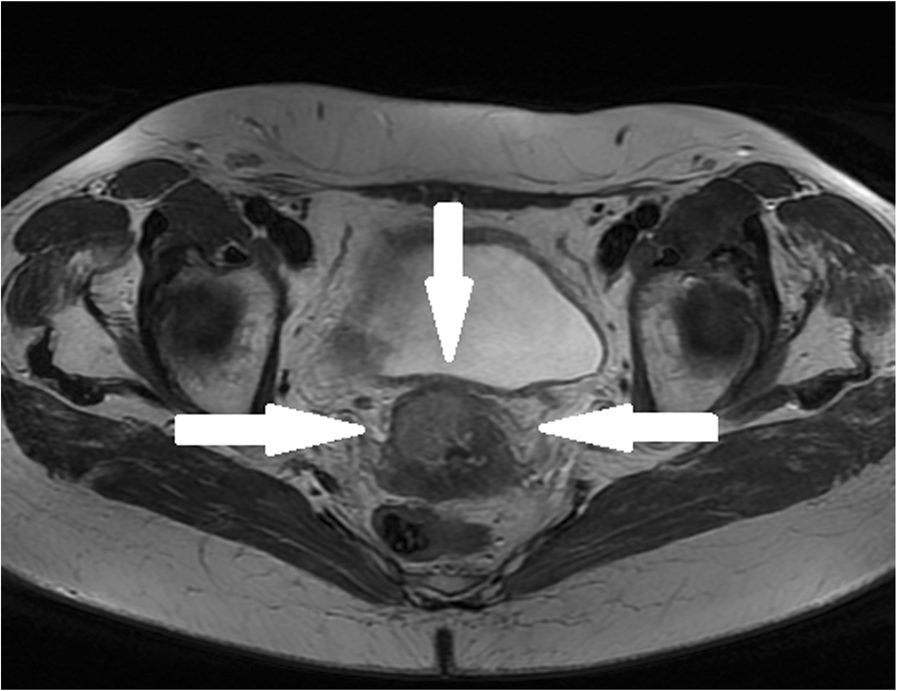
Magnetic resonance imaging showing a polypous tumor in anterior labium region of the cervix, with the size of 26x24x23mm, without parametrial infiltration, without lymphadenopathy
